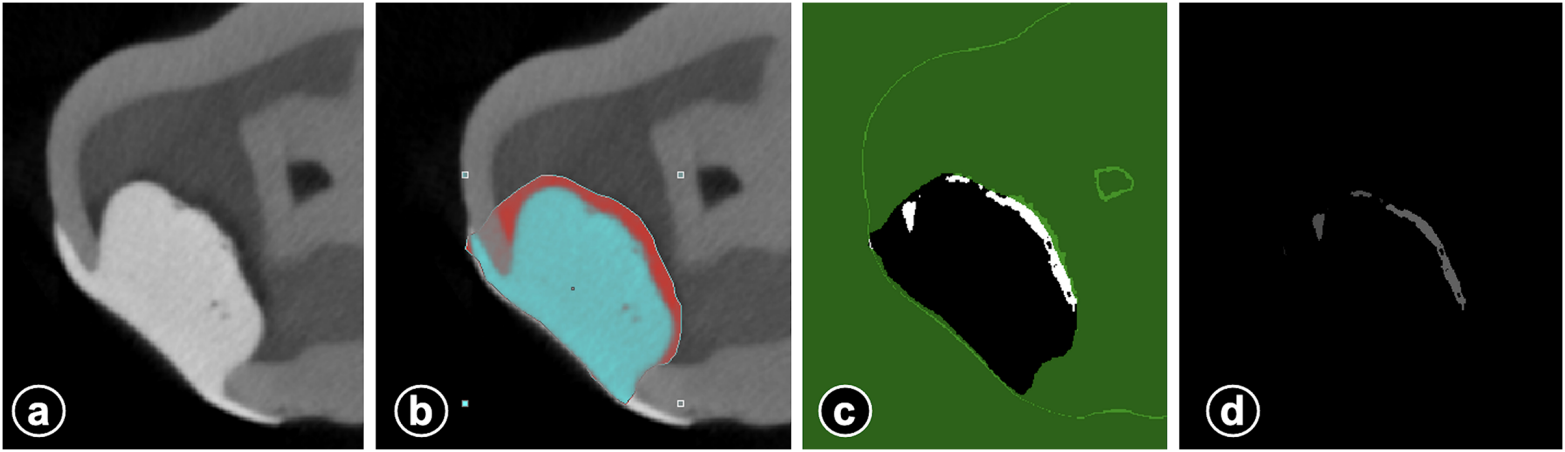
The figure series demonstrating the calculation of internal voids. Micro CT image of restorative material and voids (A) selection of ROI for determination of void volume (blue shows restorative material) (B) threshold segmentation of void and subtraction of restorative material (C) and void volume for automatic calculation (D).
